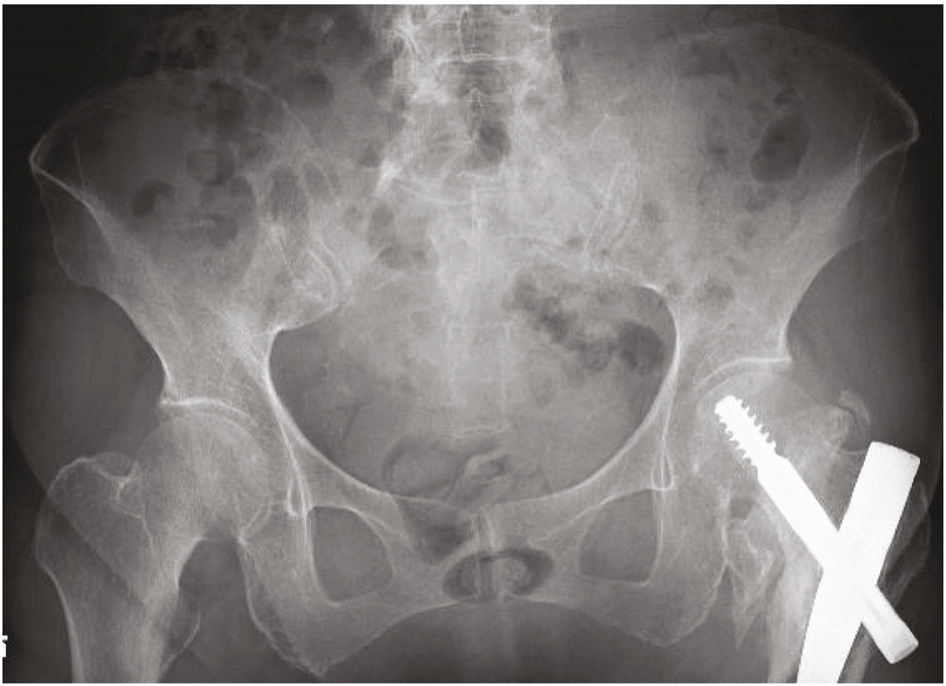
Anteroposterior pelvis radiograph.
